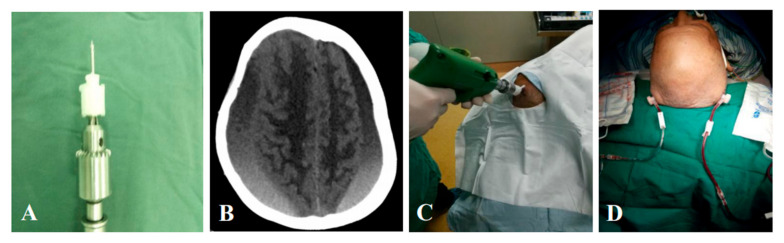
Operation procedure of YL-1 puncture needle. (A) Fixation of the YL-1 puncture needle in an electric trepanning drill. (B) Preoperative CT scanning. (C) Drilling into the hematoma cavity. (D) Postoperative drainage.
